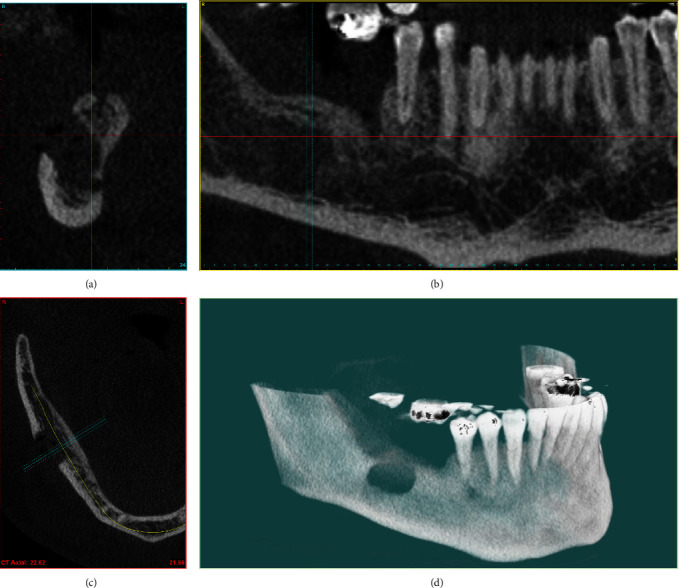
CBCT after implant removal. (a) Sagittal reconstruction. (b) Reformatted panoramic image. (c) Axial image. (d) 3D reconstruction.
